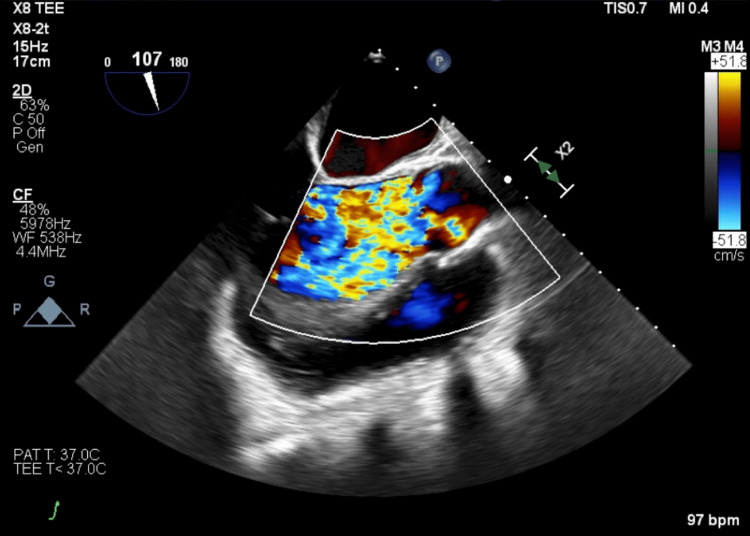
Transesophageal echocardiogram: 2D mid-esophageal long axis view at end-diastole with color-flow Doppler showing visually severe central aortic insufficiency
